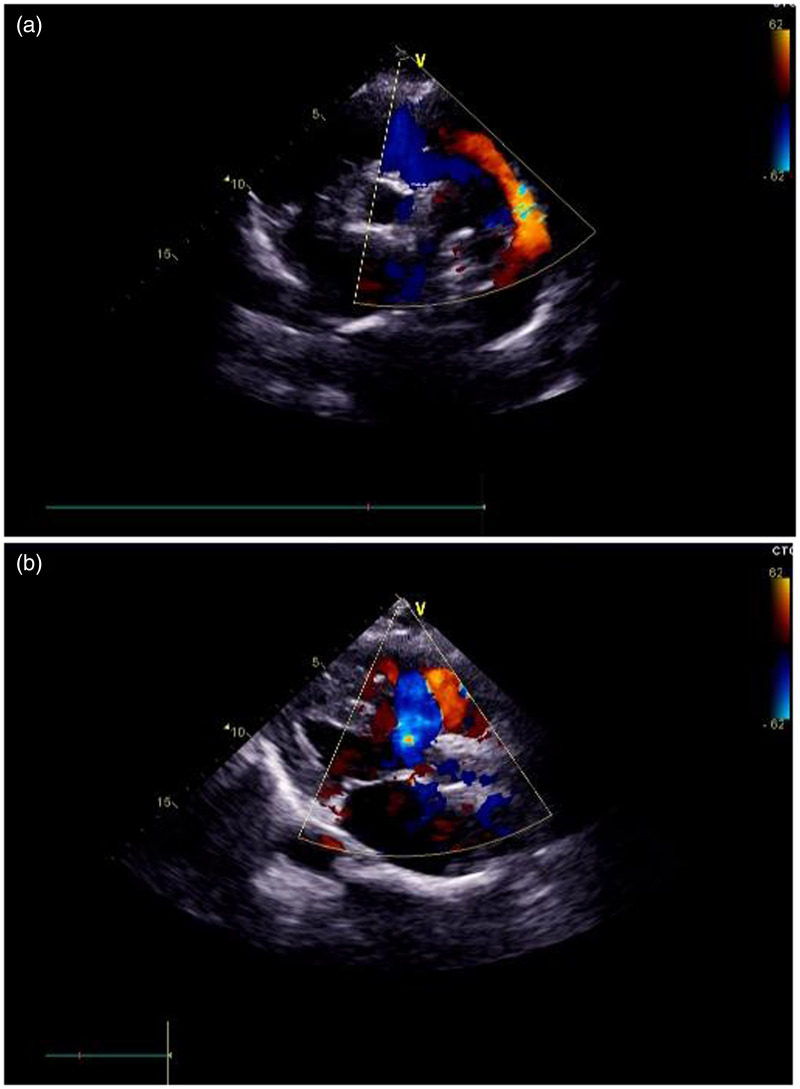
Echocardiographic images. (a) Enlargement of the left atrium and left ventricle and an abnormal conduit connecting the left pulmonary artery and descending aorta can be seen. (b) This image shows the morphology of the aortic valve and pulmonary valve, the morphology of the mitral valve and tricuspid valve, and interruption of echo in the interventricular septum.
